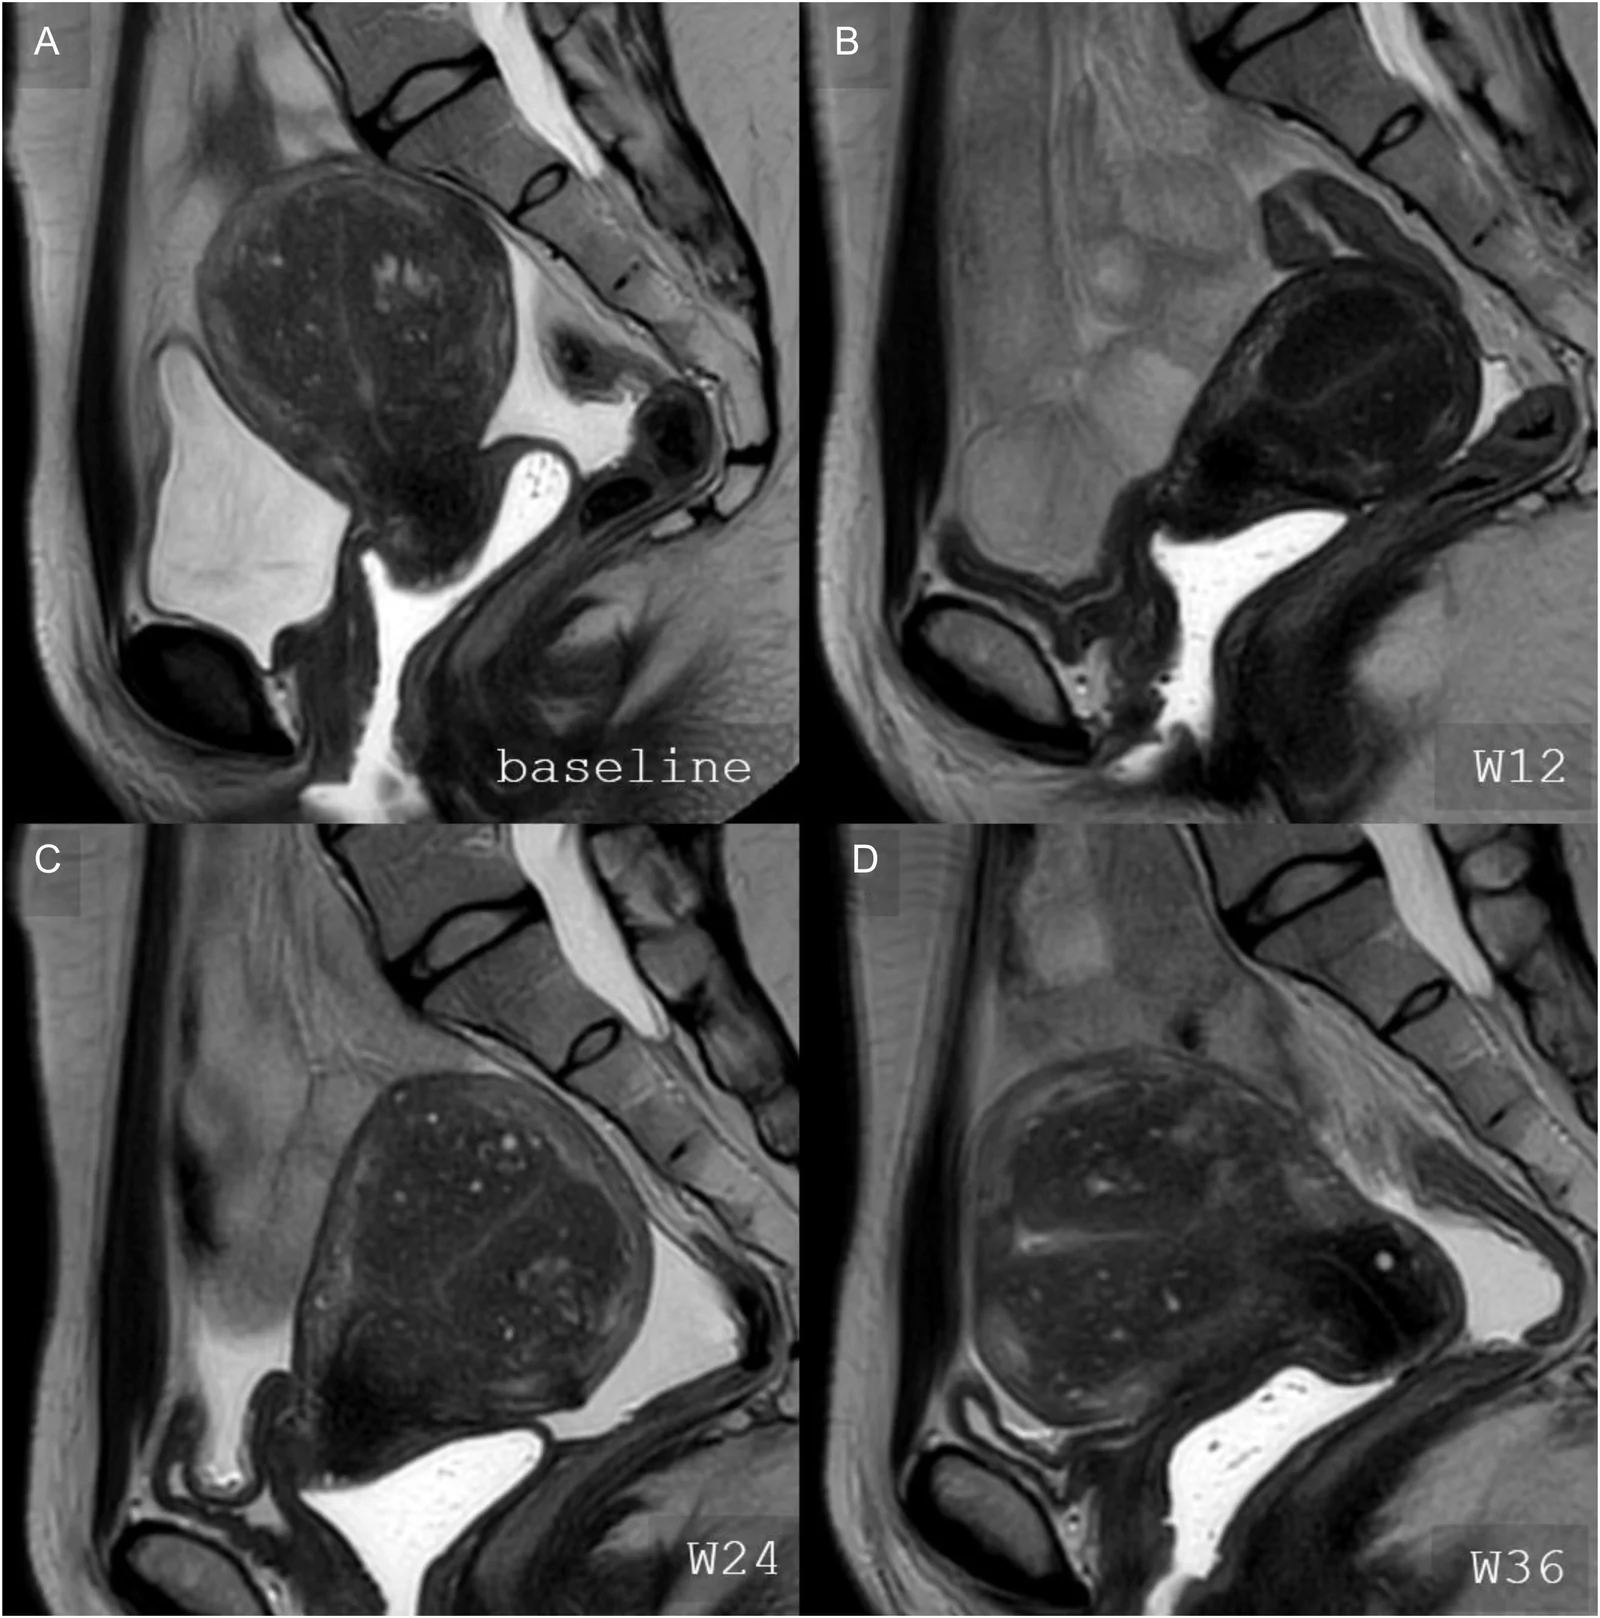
Patient 1. (A) MRI showing an enlarged uterus with diffuse and disseminated adenomyosis at baseline. (B) After 12 weeks of GnRH antagonist therapy (200 mg linzagolix, QD), a significant reduction was observed in uterine size and adenomyotic lesions. (C) The patient was then switched to 100 mg/day linzagolix, causing some regrowth, but the volume remained smaller than at baseline. (D) By week 36 (12 weeks after the end of therapy), uterine volume values returned to baseline values.
